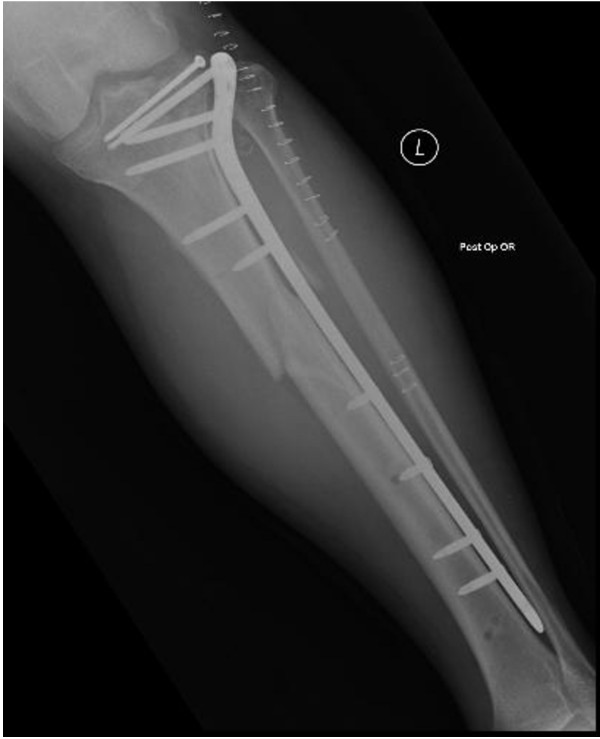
Postoperative radiograph following fixation of tibia fracture.
